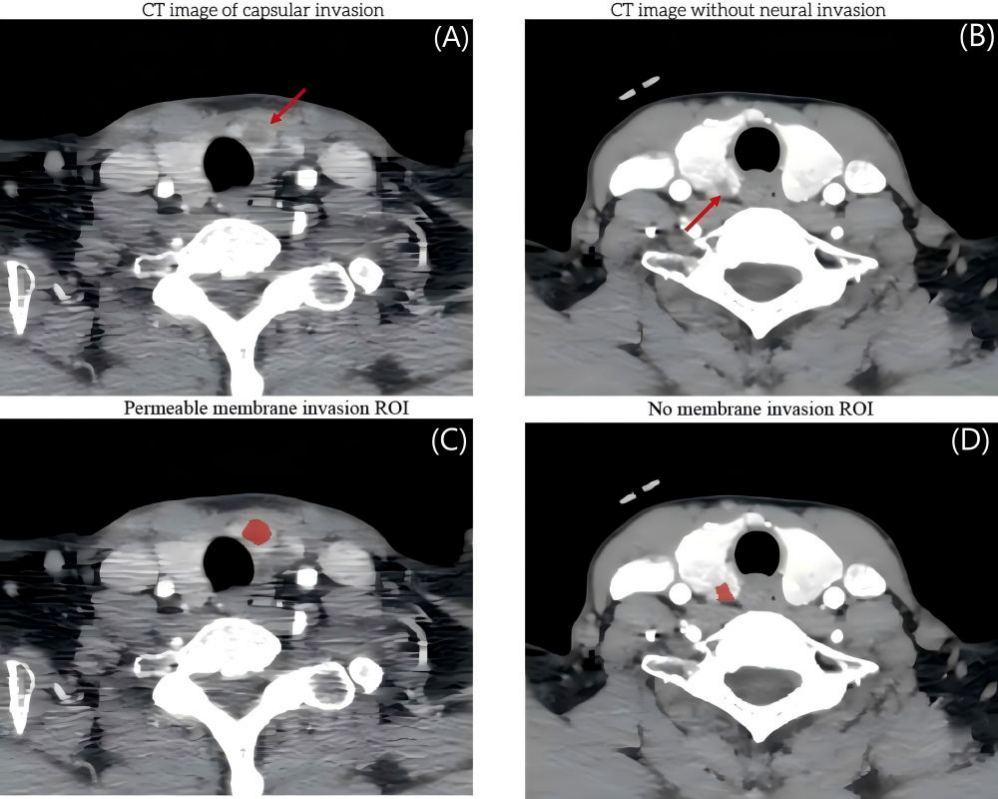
Representative arterial-phase CT images of thyroid carcinoma. (A-C) A case with pathologically confirmed capsular invasion showing irregular tumor margins (arrow). (B-D) A case without NI demonstrating well-defined tumor boundaries. CT: computed tomography; NI: neural invasion; ROI: regions of interest.
